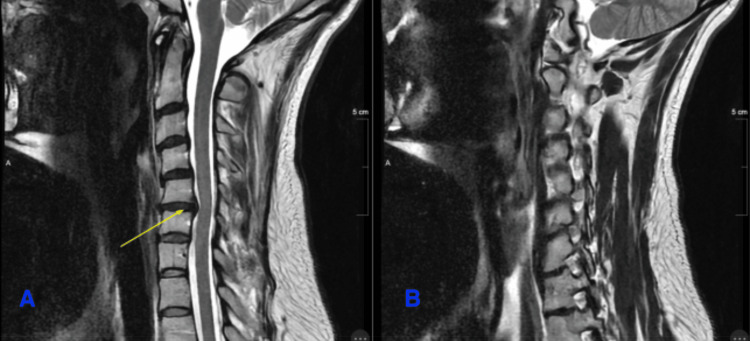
MRI of the cervical spine: T2 sagittal images (A) A moderate disc bulge posteriorly abutting the spinal cord at the C5-C6 level (yellow arrow) without any cord edema. (B) No neuroforaminal compromise at any level.
